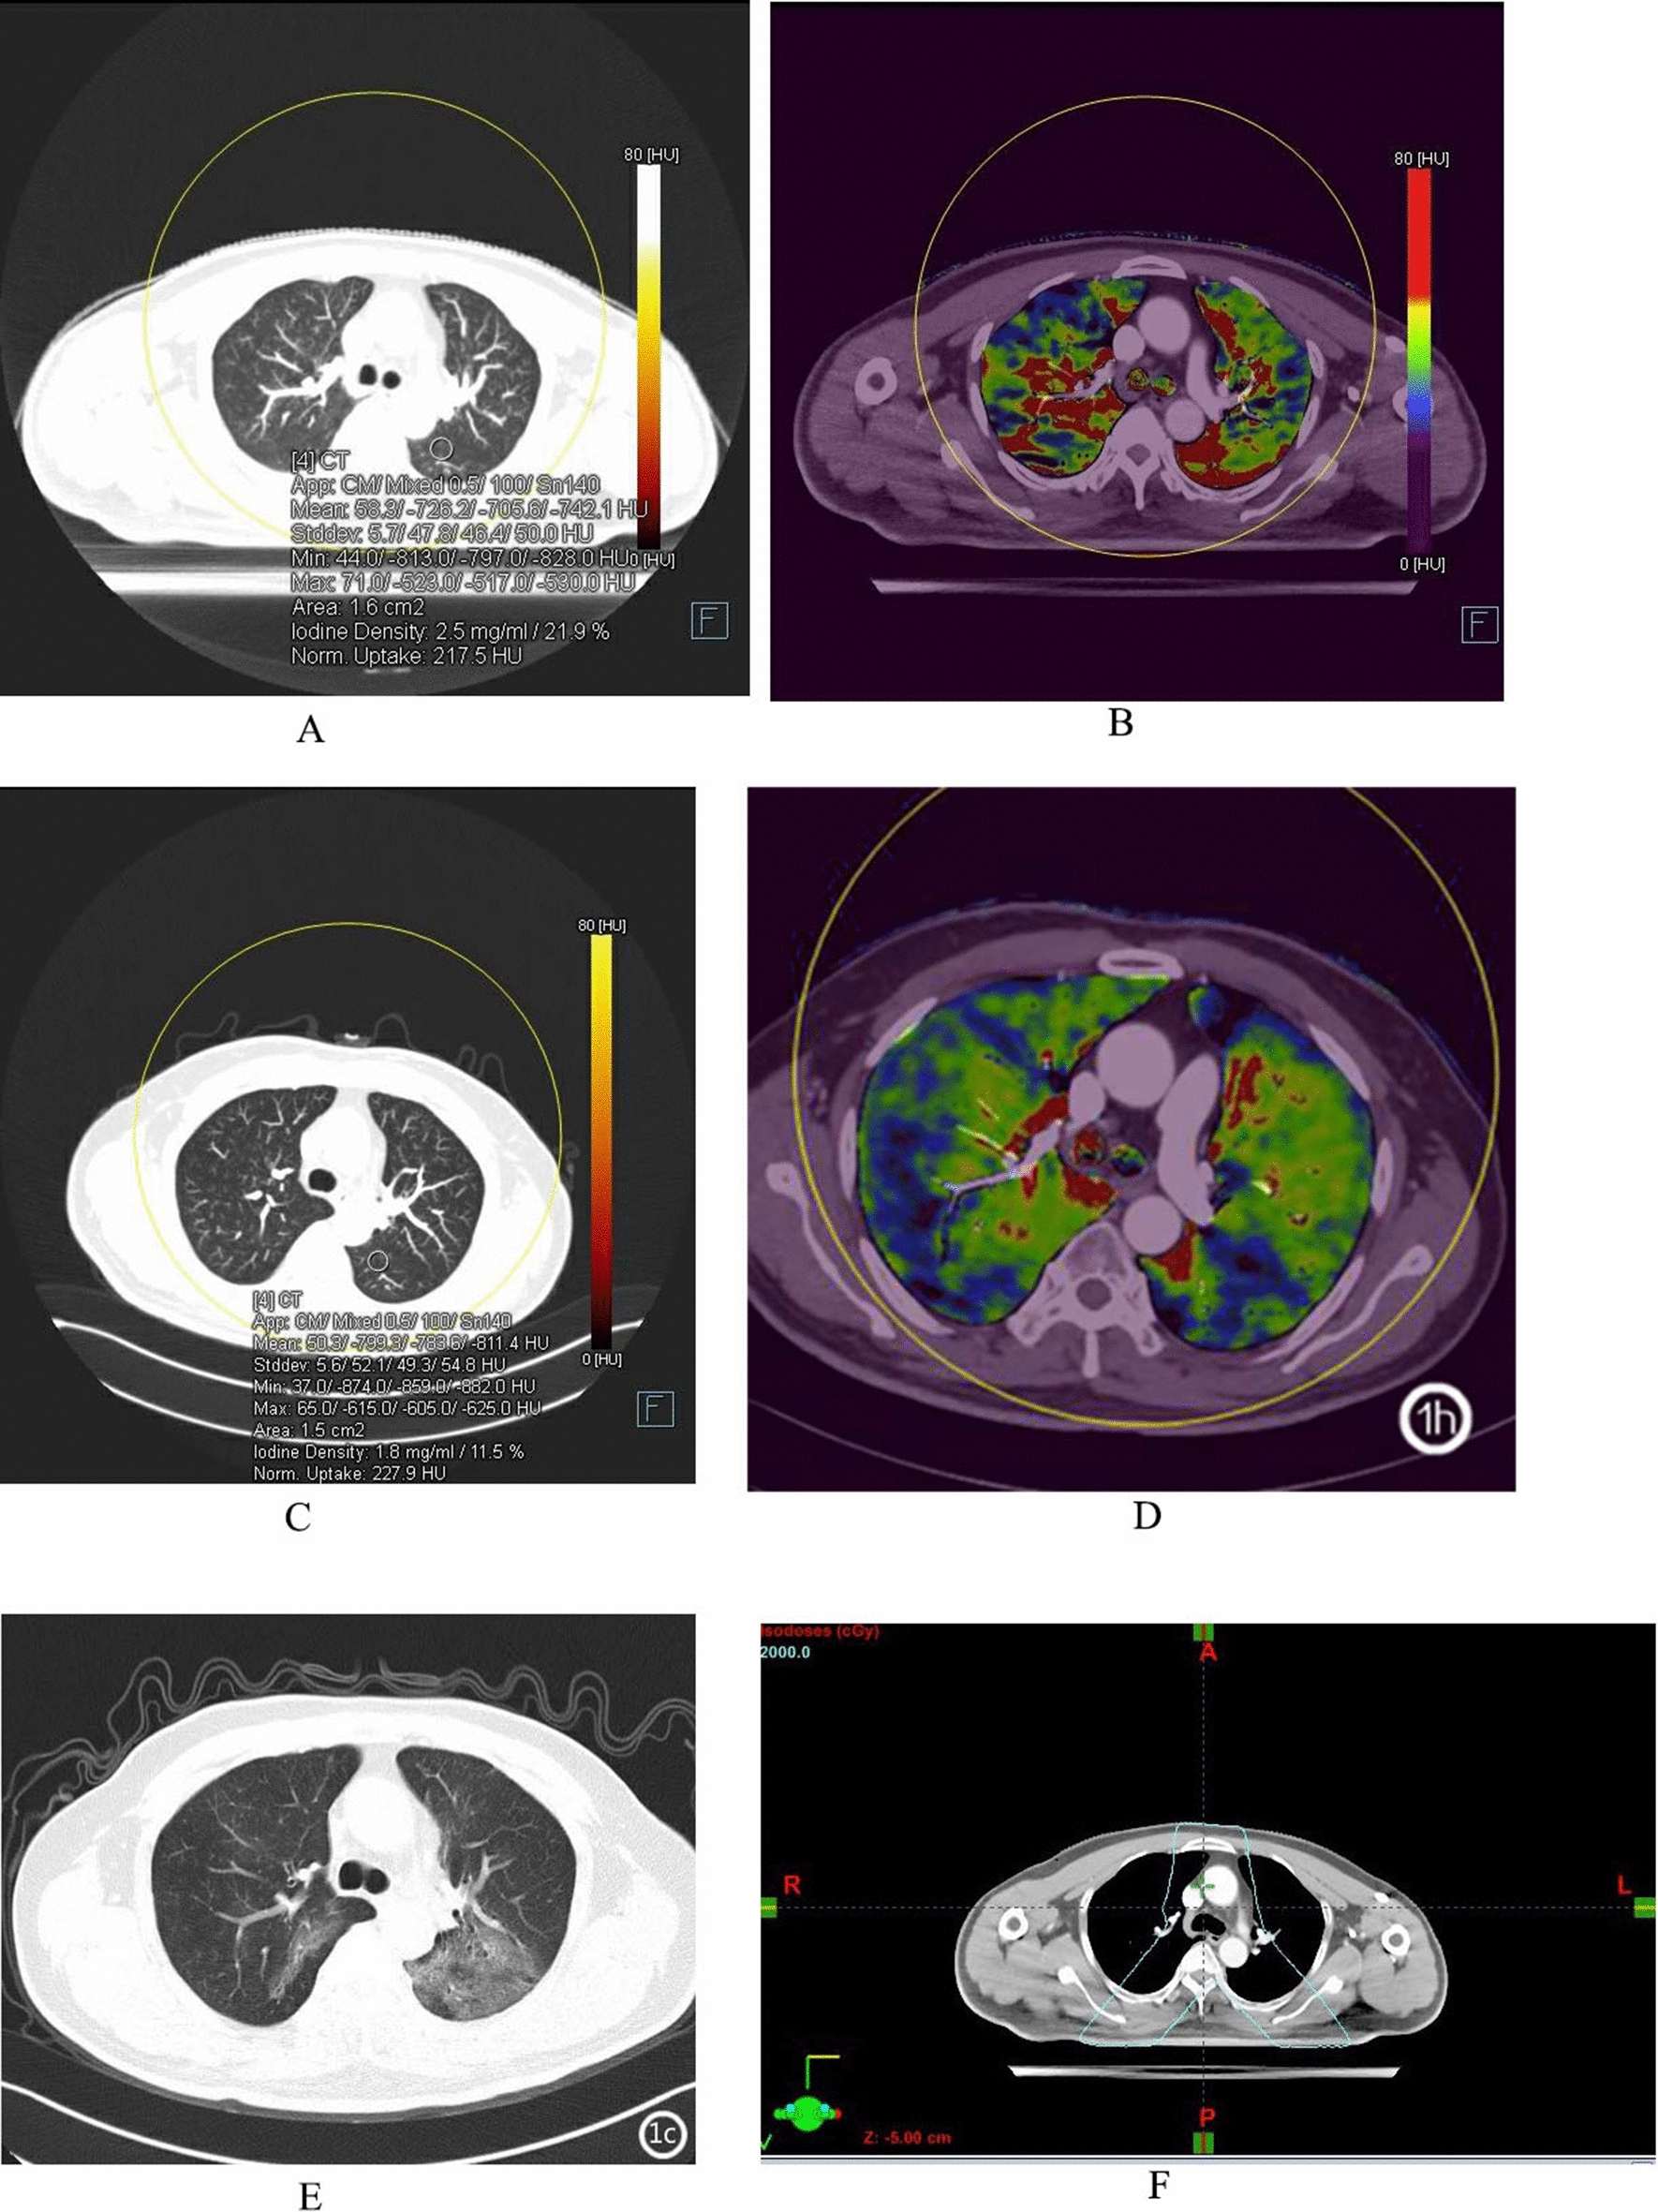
Male/60 years old, upper segment esophageal cancer; a Dual-energy lung window image before radiotherapy, the NIC value of the left lung is 0.219; b Lung PBV color image before radiotherapy, blood flow (red part) is more abundant in bilateral irradiated fields; c Dual-energy lung window image after radiotherapy, the NIC value of the left lung is 0.115; d Lung PBV color image after radiotherapy, blood flow (red part) is less than before; e CT image during follow-up period, ARP is seen in both lungs; f TPS transverse localization image, the region of V_>20 Gy_ irradiation is within the green line, and ARP is seen in the region
